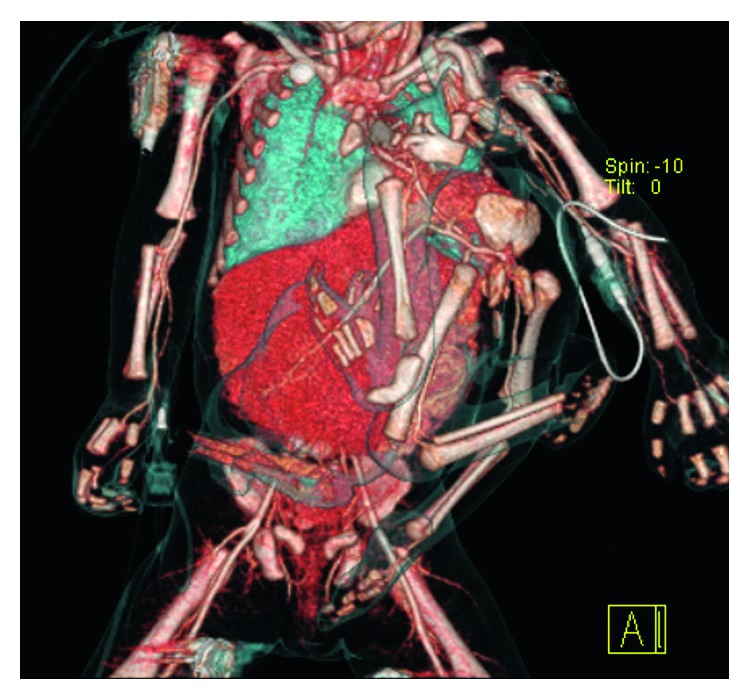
Three-dimensional CT reconstruction of presurgical presentation. Note the ventral vessel deriving from the aortic arch pulling into the parasite and the retrograde proceeding vessel into the autosite via the omphalocele as in the falciform ligament.
